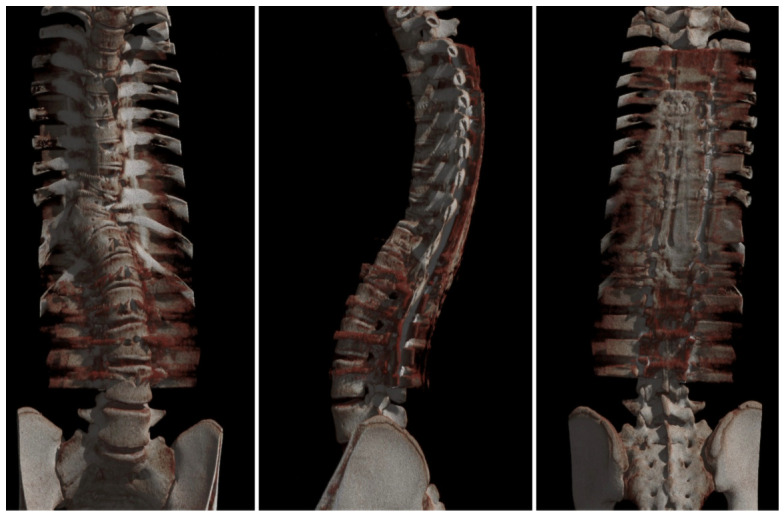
The imaging an CT obtained at a 2-year of follow-up showed posterior spinal fusion.
